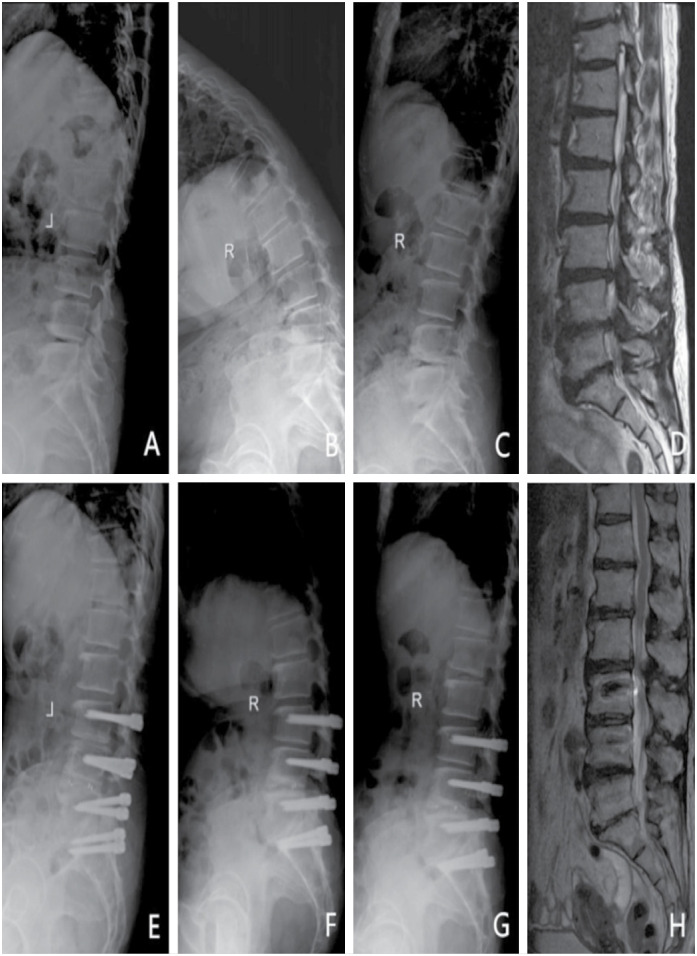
A 58-year-old female patient underwent surgery with a hybrid fixation system due to lumbar disc herniation and spinal stenosis in L3/4, L4/5, and L5/S1. (A) Pre-operation lateral X-ray. (B–C) Pre-operation flexion and extension X-ray, the ROM of operating segments was 40.0°, and that of the upper adjacent segment was 7.9°. (D) Pre-operation T2WI MRI demonstrated L3/4, L4/5, and L5/S1 disc herniation. (E) Lateral X-ray at 43 months after surgery. (F–G) Flexion-extension X-ray at 43 months after surgery, the ROM of operating segments was 2.6°, and that of the upper adjacent segment was 10.5°. (H) T2WI MRI at 43 months after surgery.
